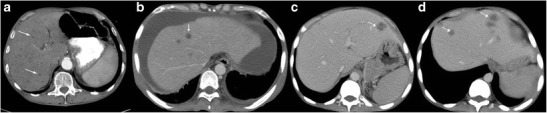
a Axial CT image of a 45-year-old woman with disseminated tuberculosis, showing multiple tiny hypodense lesions (*thin arrows*) in both lobes of the liver. b Disseminated disease in a 25-year-old man with multiple low attenuation cystic appearing lesions (*short arrows*) in the right lobe of the liver and associated ascites. c, d Contrast CT in a middle-aged woman with fever and disseminated tuberculosis exhibiting multiple hypodense lesions with subtle peripheral enhancement (*dashed arrows*)
